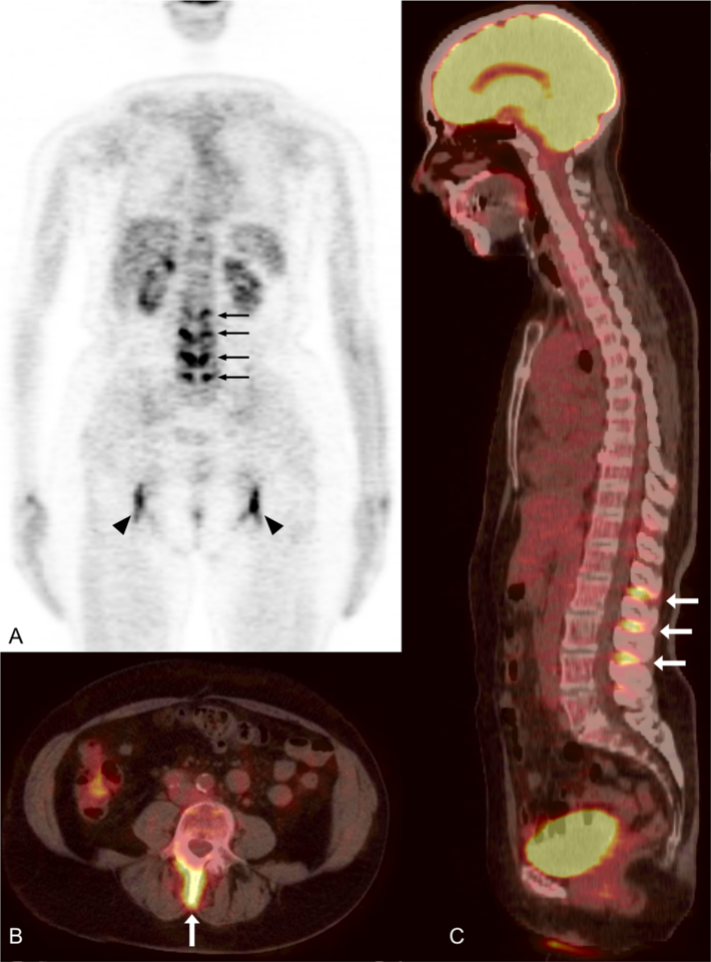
FDG uptake in the lumbar interspinous spaces of a patient with polymyalgia rheumatica. (A) Coronal PET image; (B) axial PET/CT section at the level of the 3^rd^ lumbar vertebra; (C) sagittal PET/CT image showing interspinous bursitis (arrows) between 1^st^ and 2^nd^, 2^nd^ and 3^rd^, 3^rd^ and 4^th^ lumbar vertebrae. FDG, 18 F-fluorodeoxyglucose; PET/CT, positron emission tomography integrated with computed tomography.
